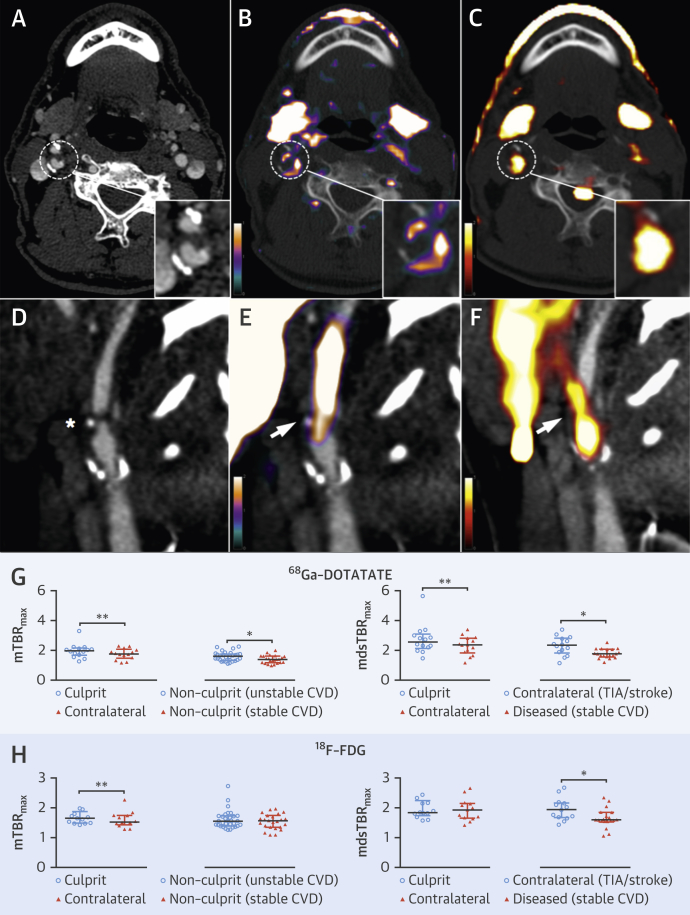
Carotid PET Inflammation Imaging: TIA/Stroke Views from a 66-year-old man ([top] axial plane) and a 70-year-old man ([bottom] sagittal plane), both of whom had TIAs resulting from right internal carotid artery lesions, shown on CT (A [hatched circle], D [∗]), with intense culprit plaque inflammation (hatched circles/arrows) detected by ^68^Ga-DOTATATE (B, E) and reproduced by [^18^F]FDG (C, F). Graphs compare culprit versus nonculprit ^68^Ga-DOTATATE (G) and [^18^F]FDG (H) TBR_max_ values (^68^Ga-DOTATATE: culprit n = 14; contralateral n = 14; nonculprit [unstable CVD] n = 31; nonculprit [stable CVD] n = 24; contralateral [TIA/stroke] n = 14; diseased stable CVD n = 19; [^18^F]FDG: culprit n = 13; contralateral n = 13; nonculprit [unstable CVD] n = 31; nonculprit [stable CVD] n = 24; contralateral [TIA/stroke] n = 13; diseased [stable CVD] n = 19). TIA = transient ischemic attack; other abbreviations as in Figures 1, 2, and 3.
